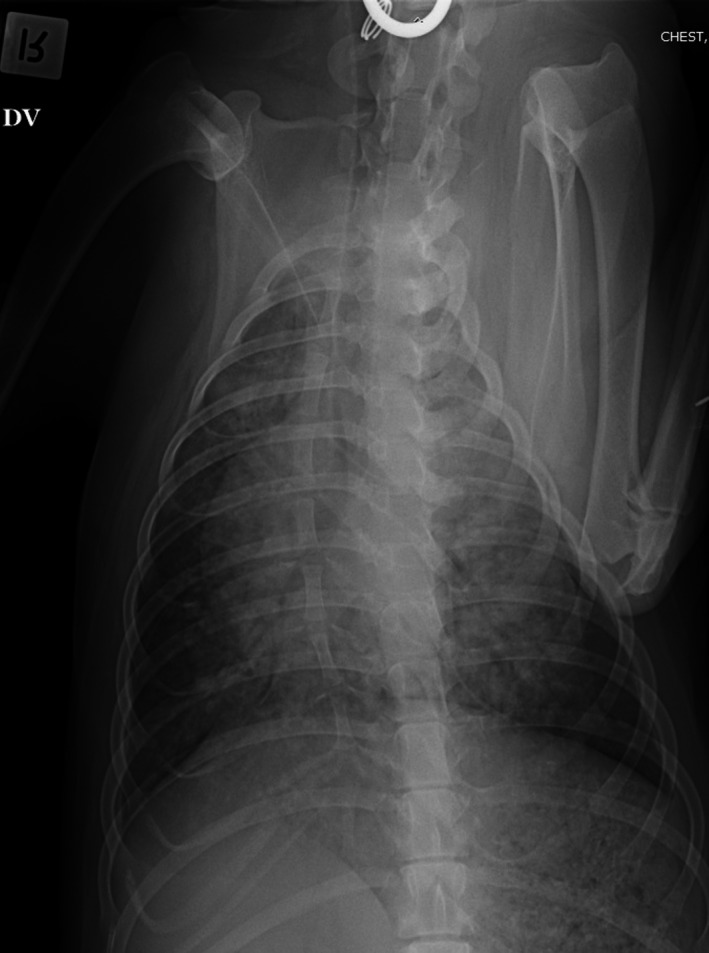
Dorsoventral thoracic radiograph obtained at Day 11
